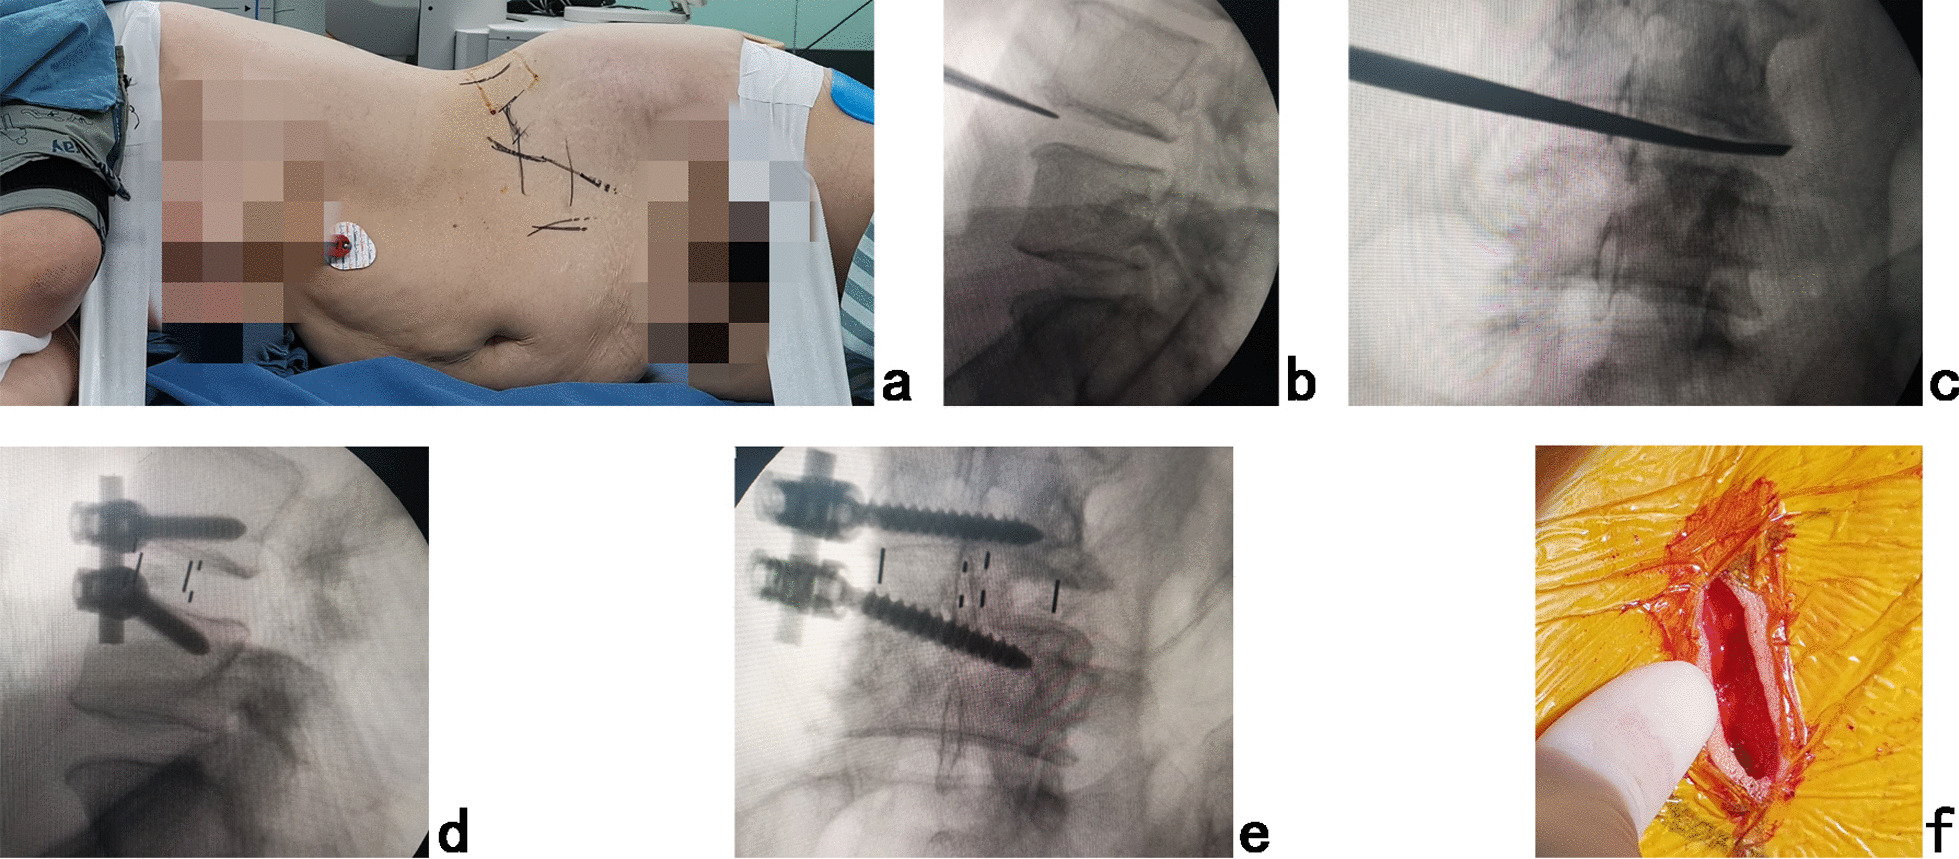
a Patient was placed into right lateral decubitus position for mini-incision OLIF and anterolateral screws rod fixation under general anesthesia. The target intervertebral space was positioned using b C-arm view. The spatula was inserted into intervertebral space to cut the contralateral fibrous annulus, which was confirmed by c X-ray image. After the OLIF cage was placed into disc space parallelly to the endplate and anterolateral screws rod fixation was performed, d lateral and e posteroanterior C-arm view confirmed good position of internal instruments. f The picture showed the mini-incision of OLIF
